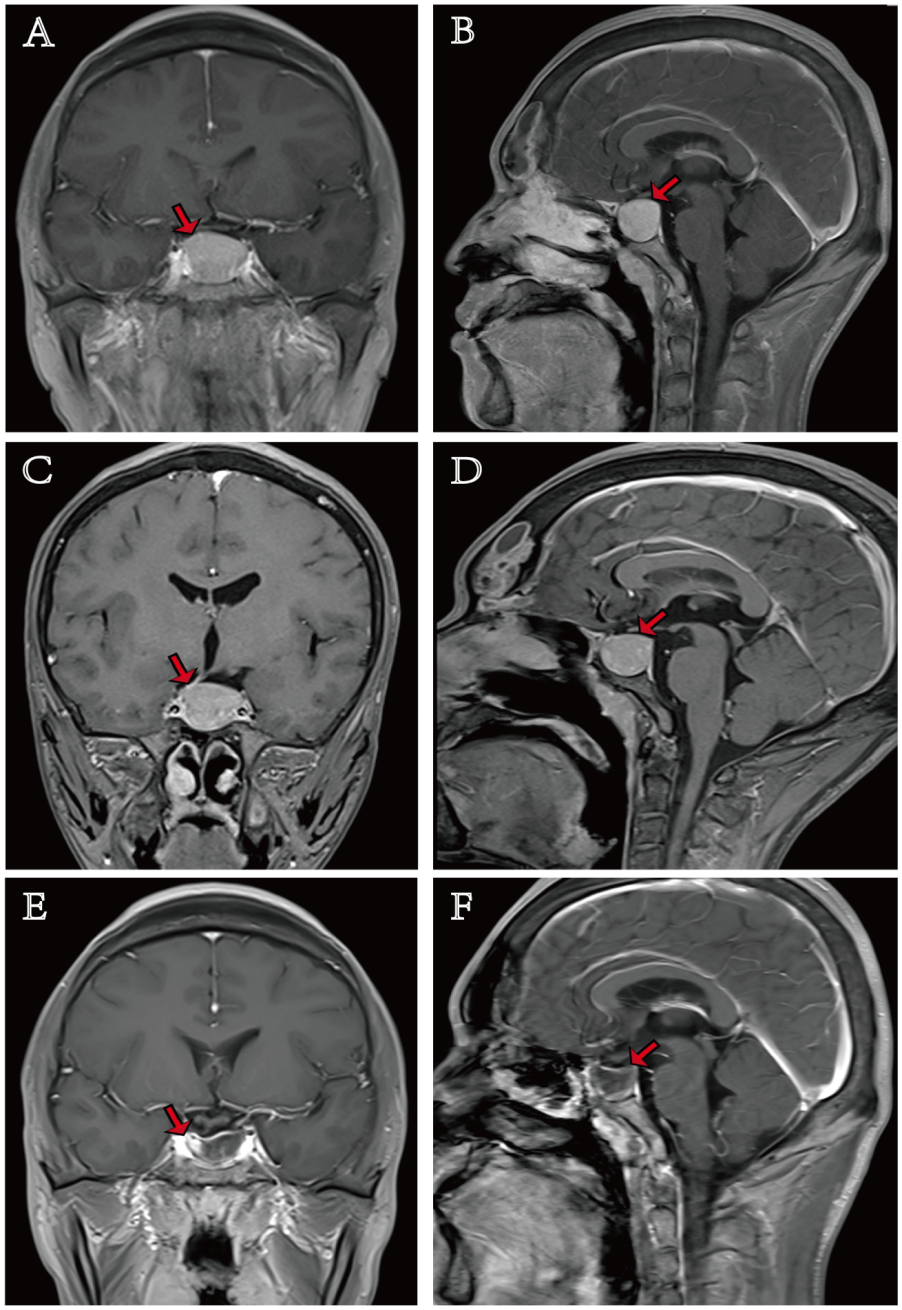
Serial contrast-enhanced pituitary magnetic resonance imaging (MRI). The initial MRI pituitary scan revealed a pituitary macroadenoma (19 × 25 × 23 mm). Initial diagnosis: (A) axial view and (B) sagittal view; 1 year after initial diagnosis: (C) axial view and (D) sagittal view; 3-month postoperative follow-up: (E) axial view and (F) sagittal view.
